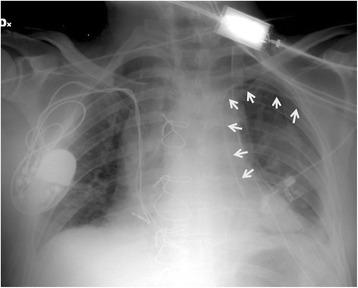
Post-operative X-ray showing the central venous catheter descending on the left side of the mediastinum (arrows)
